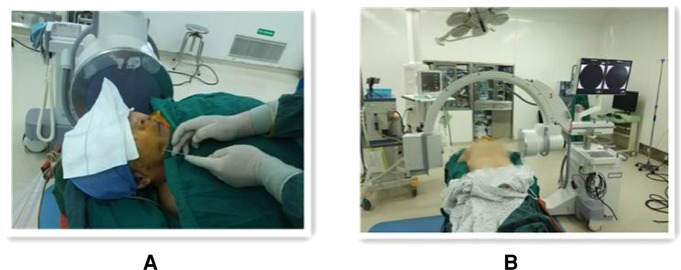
Operating procedure of percutaneous balloon compression guided by the C-arm set x-ray machine alone. (A) Freehand puncture with puncture needle at approximately 2.5 cm marker point next to the corner of the mouth on the painful side. (B) Showing the spatial layout between the patient, C-arm x-ray machine.
